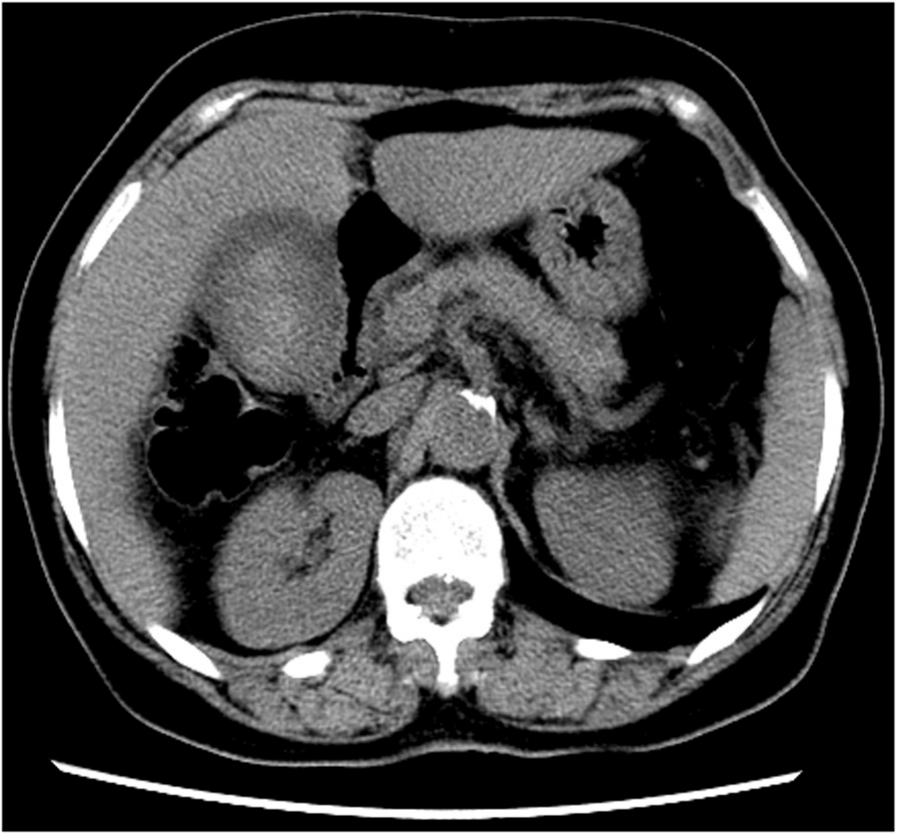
Plain computed tomography (CT) abdomen showing gallbladder grossly distended and soft tissue mass in the gallbladder.
